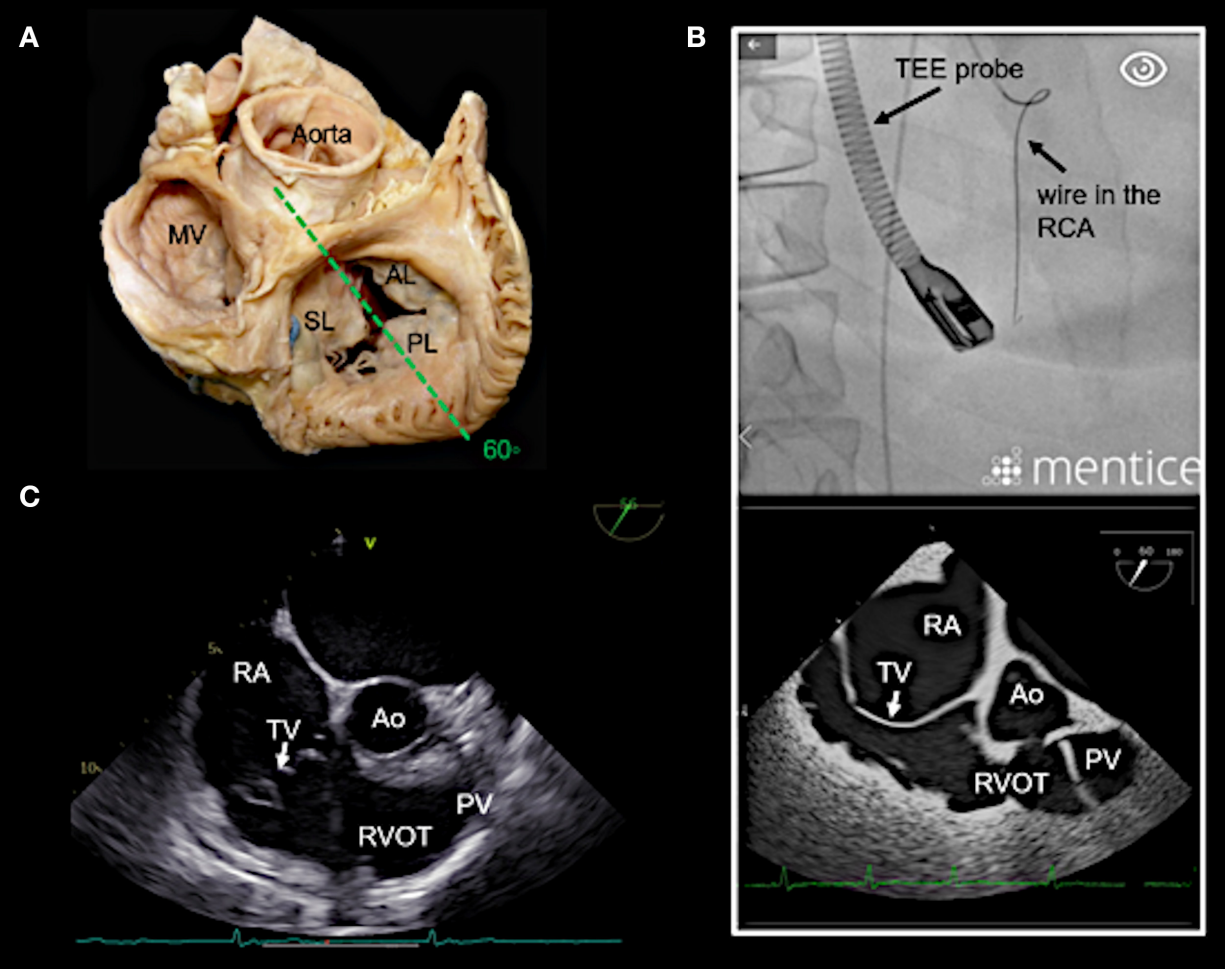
Right ventricular (RV) inflow-outflow tract view (50–70°) as seen in mid- to deep esophageal views. (A) The imaging plane for the RV inflow-outflow tract is found here in a deeper transesophageal view at 60° (see also Figure 3A). (B) The upper picture shows the positioning of the TEE probe in fluoroscopy (35° RAO, 6° CRANIAL). (C) A 2D TEE RV inflow-tract view is shown (slightly higher mid-esophageal position of the TEE probe). Ao, aorta; RVOT, right ventricular outflow tract; PV, pulmonary valve; other abbreviations see Figure 3.
